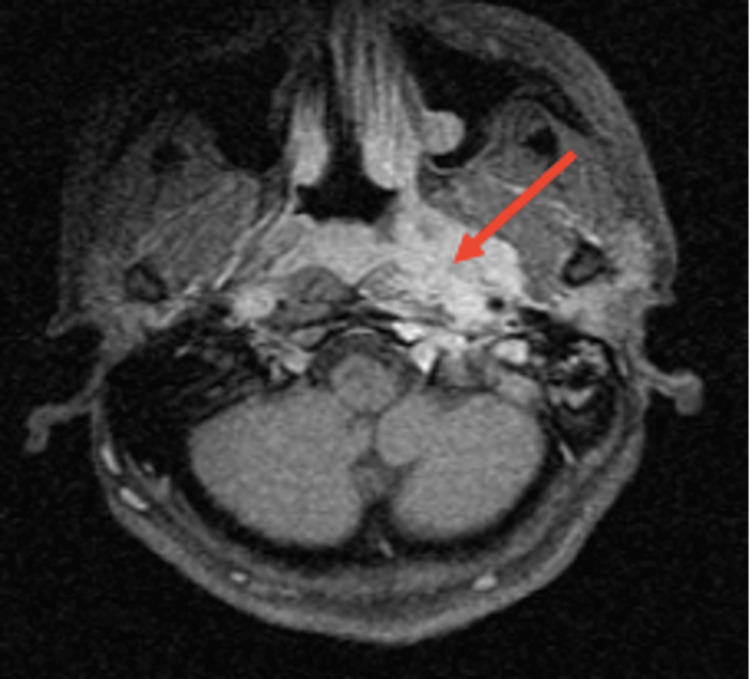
Brain MRI (axial plane, with and without 0.5 mmol/mL gadolinium) revealing a left-sided nasopharyngeal mass (arrow) MRI, magnetic resonance imaging
